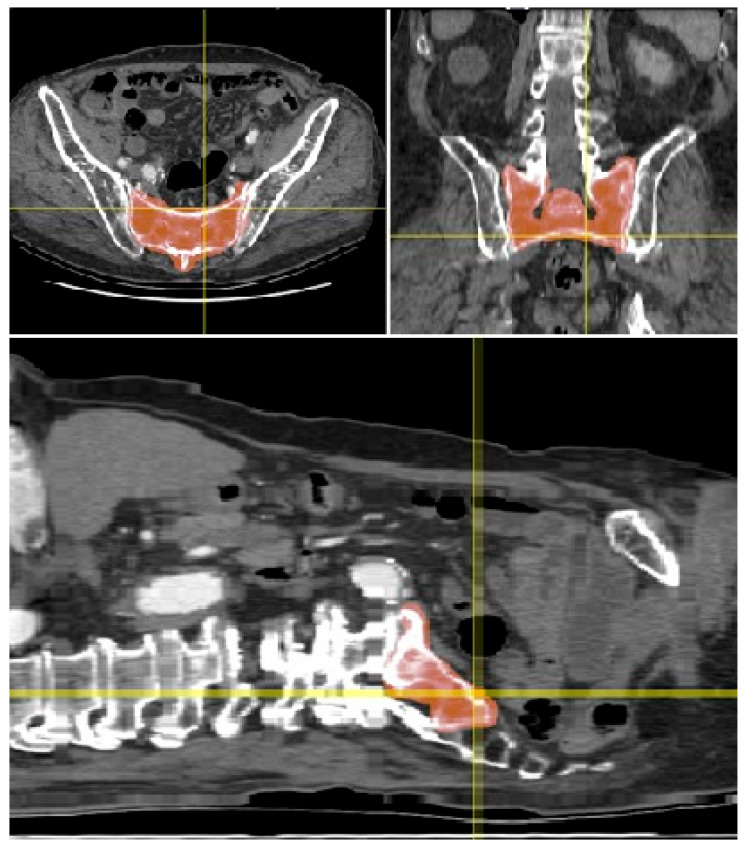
Three orthogonal section views of the patient abdominal multislice (MS) CT scan showing the sacrum (highlighted in red; plane coordinates are marked by the crosshairs). The transverse pixel sampling was 0.672 mm and the longitudinal z-spacing was 2.976 mm.
